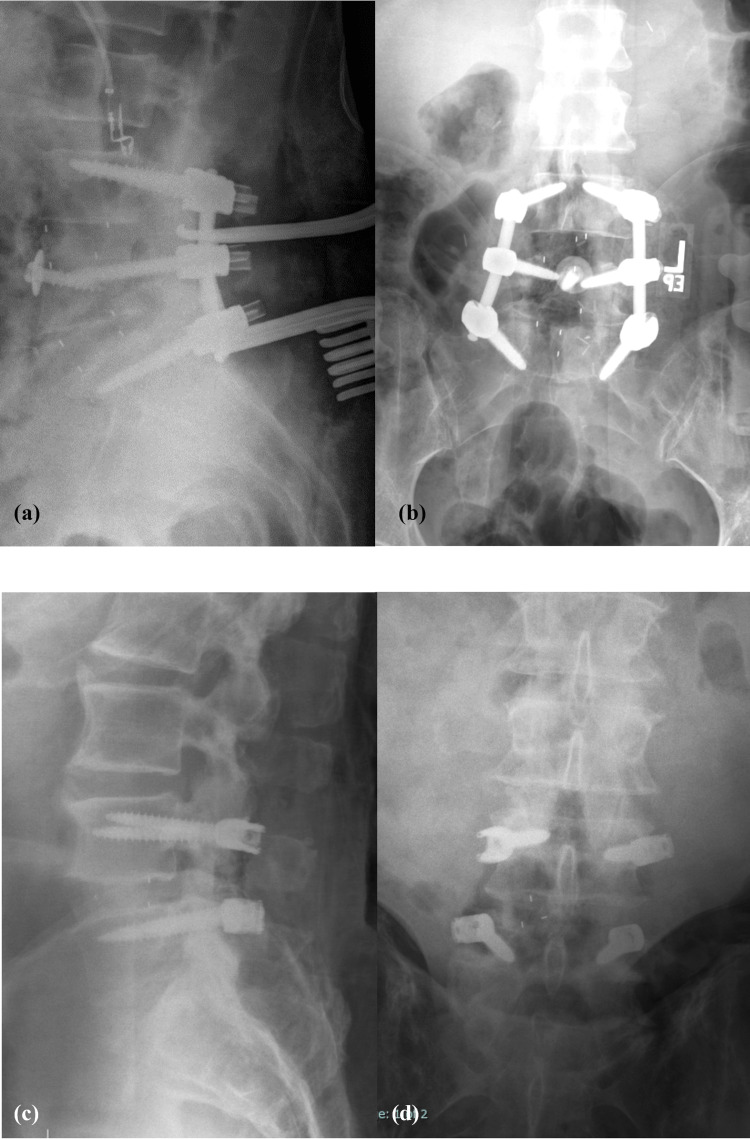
Lateral plus anteroposterior (LAT+AP) radiographs - examples of (a) false positive, LAT (subject 113); (b) false positive, AP (subject 113); (c) true negative, LAT (subject 8); and (d) true negative, AP (subject 8)
